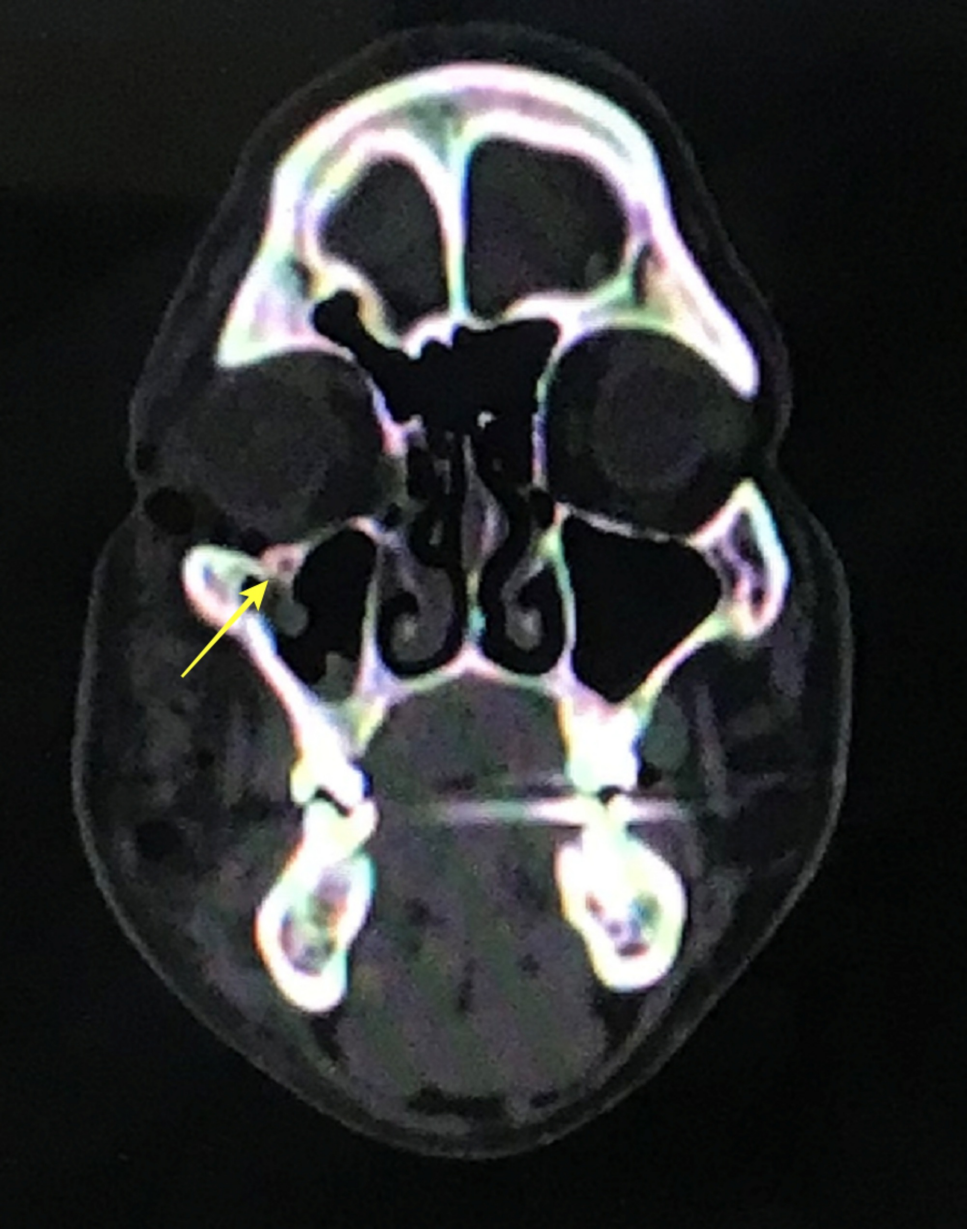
Coronal computed tomography view demonstrating fracture at the infraorbital rim
